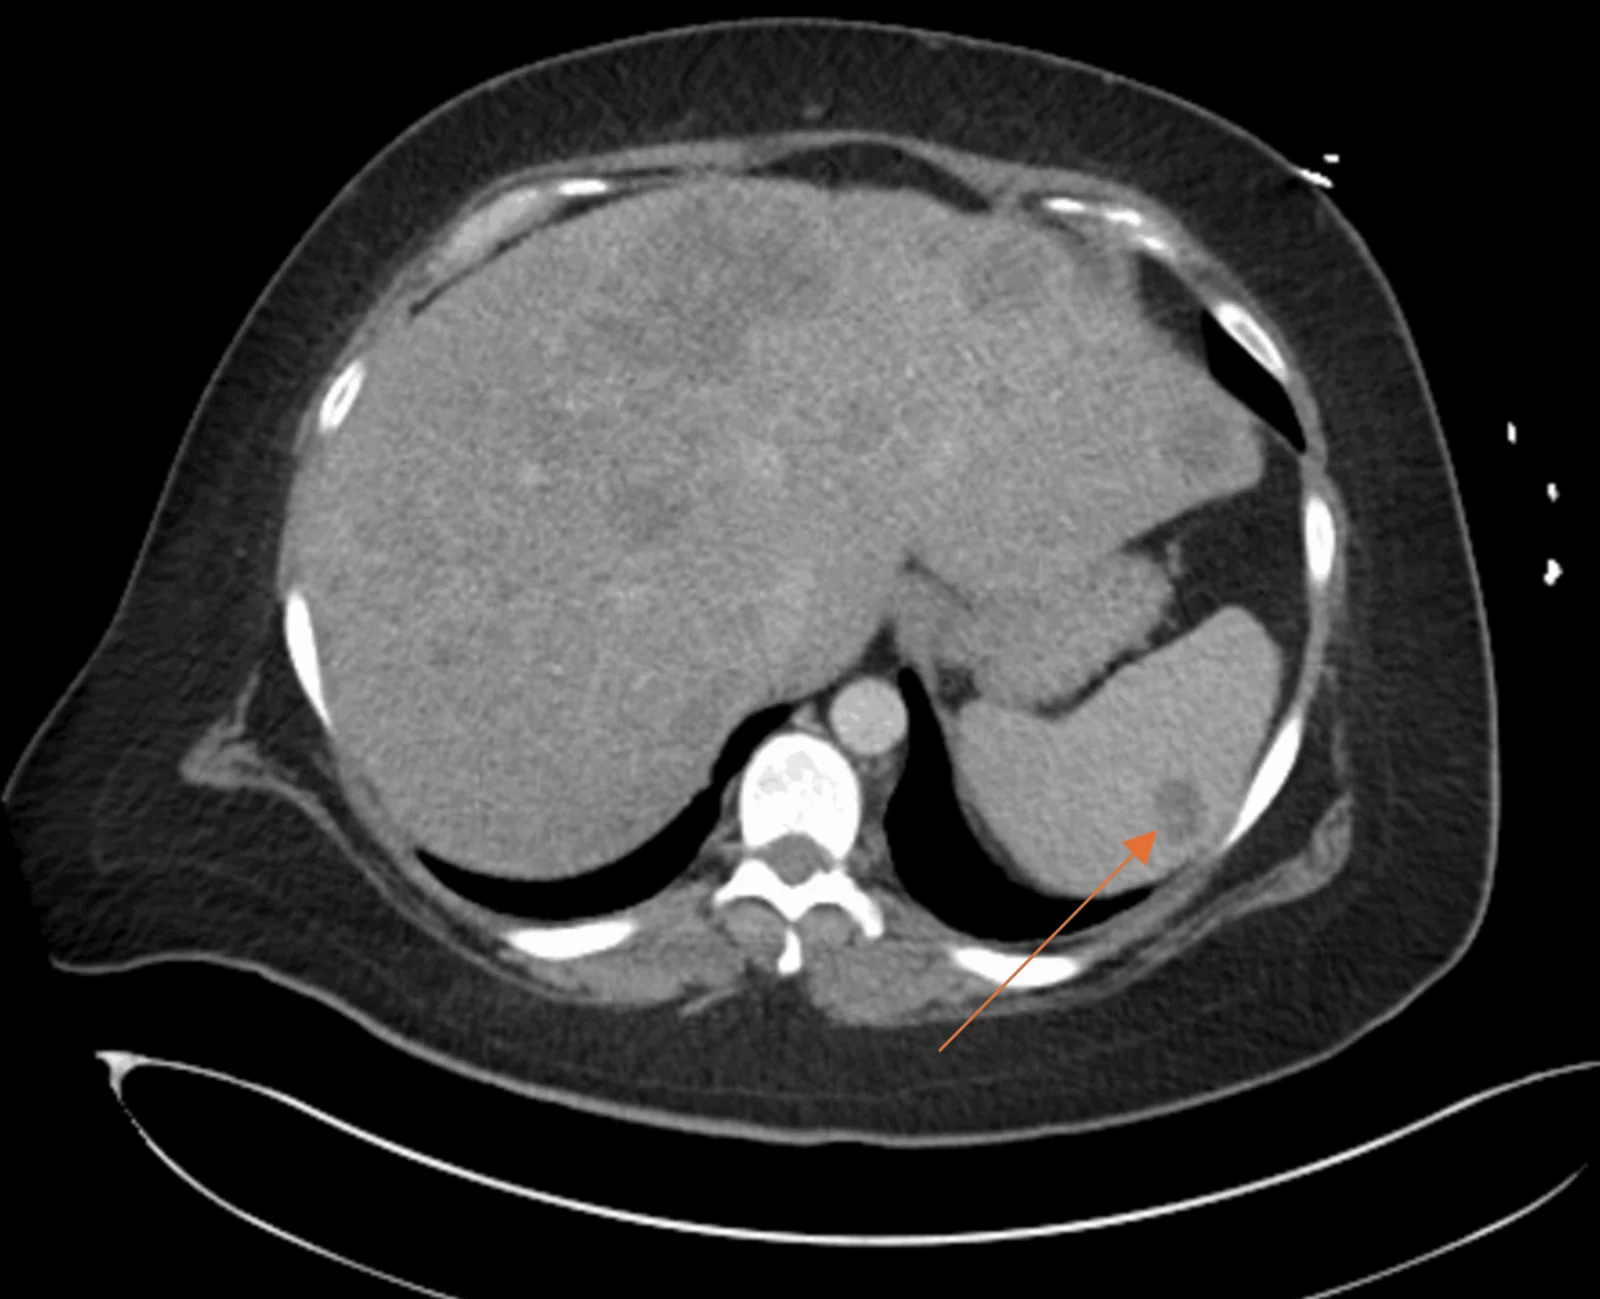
Axial CT of the abdomen and pelvis showing splenic hypodensity (arrow) as well as hepatic mass lesions
